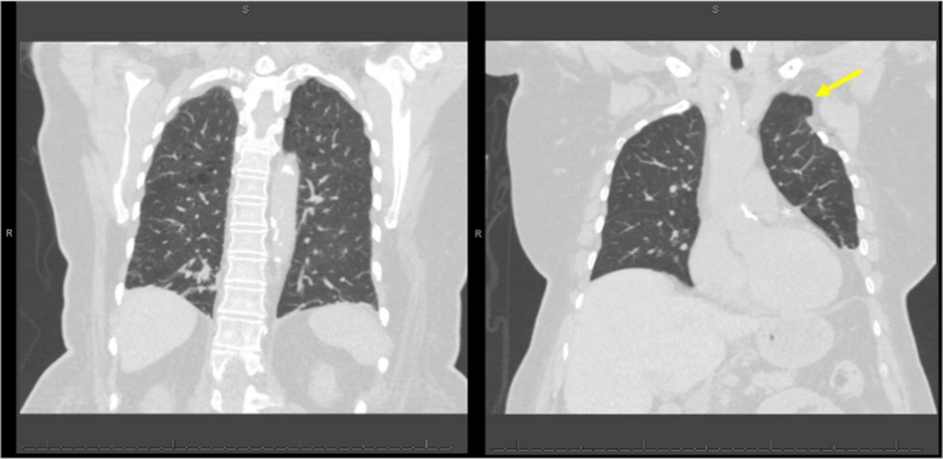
Computed tomography (CT) chest coronal view with emphysema and no evidence of pneumothorax. The yellow arrow is pointing to the left anterior apical lung herniation at the site of prior surgical first rib removal.
